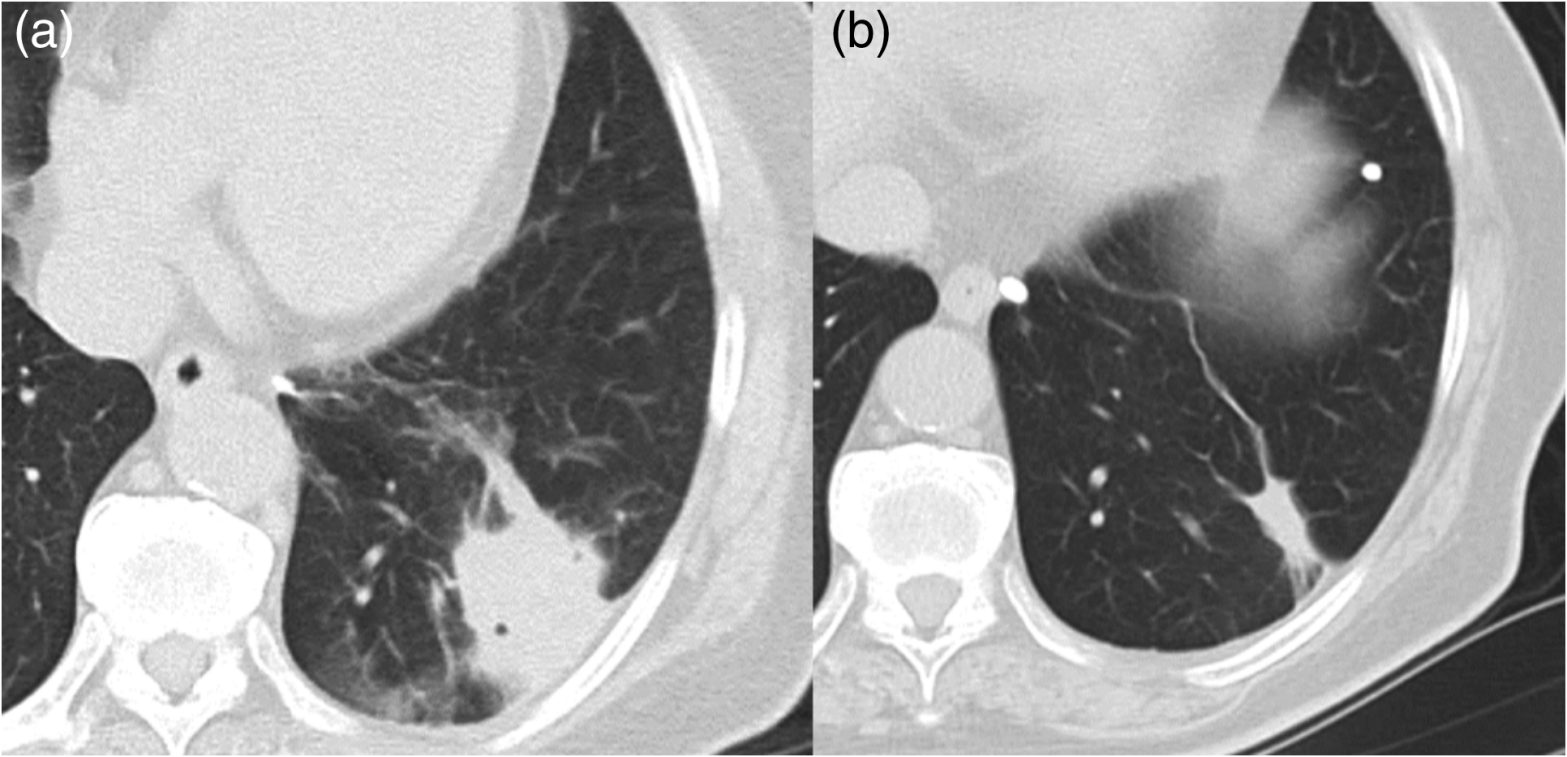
Chest computed tomography (CT) revealed consolidation before the removal of the broncholiths (A), and three months later, CT showed gradual regression of consolidation (B).
